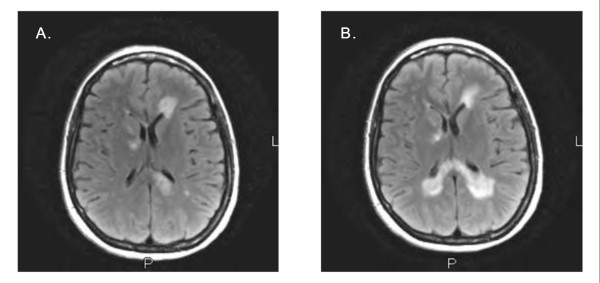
Comparison of brain MRIs between patient's first (A) and second (B) admission. A) Axial T2-flair MRI from 2 February 2009 demonstrating foci of peri-ventricular hyperintensity (more evident on the left than the right). Other findings included left greater than right lateral ventriculitis, basal meningitis, encephalitis involving the peri-ventricular and right basal ganglia white matter, and enhancing lesions in the left pons and the lower third of the medulla. B) Axial T2-flair MRI from 9 March 2009 demonstrating enlargement of prior hyperintense foci and new hyperintensity in the right posterior peri-ventricular white matter. Other findings included new enhancing lesions in the splenium of the corpus callosum, decreased enhancement in the right corona radiata and left frontal horn, and unchanged enhancements in the pons and medulla.
